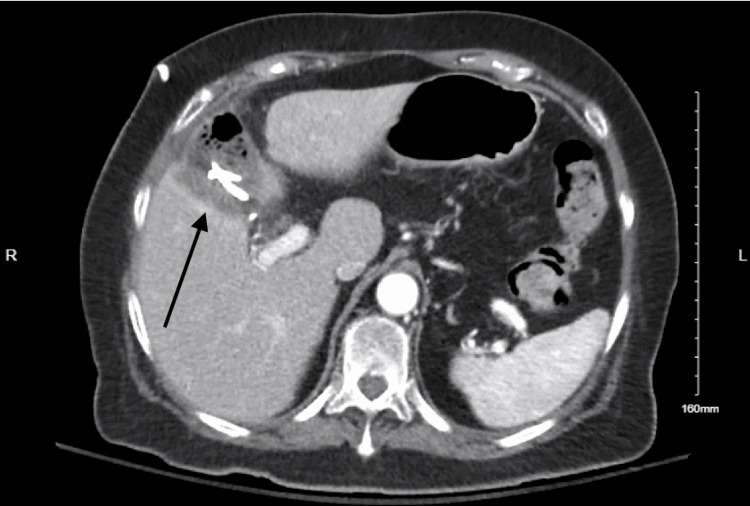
Contrast-enhanced CT scan with a drain in place, showing perihepatic fluid collection
